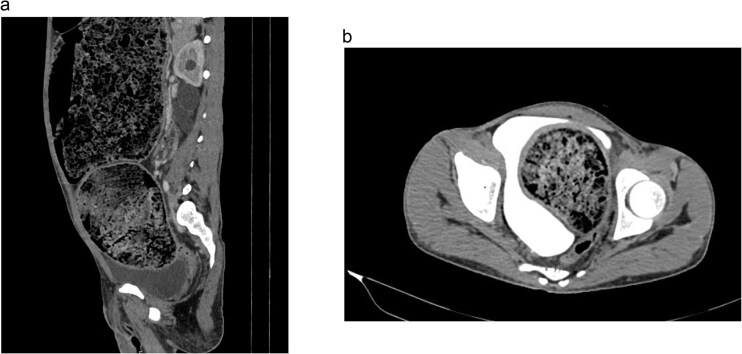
(a and b) Dilated transverse, descending and sigmoid colon in CT-abdomen.
